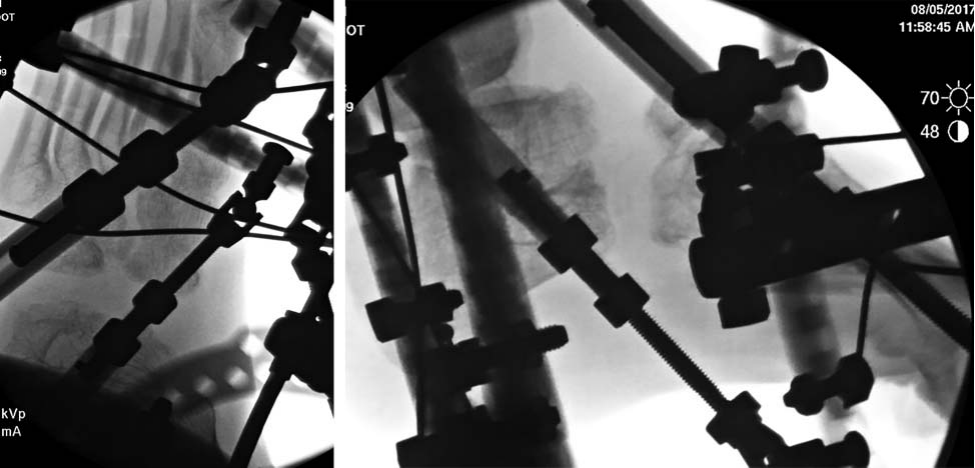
Anteroposterior and lateral radiographs (late stage 1) demonstrating gradual distraction through the Chopart joint using the hexapod fixator.
